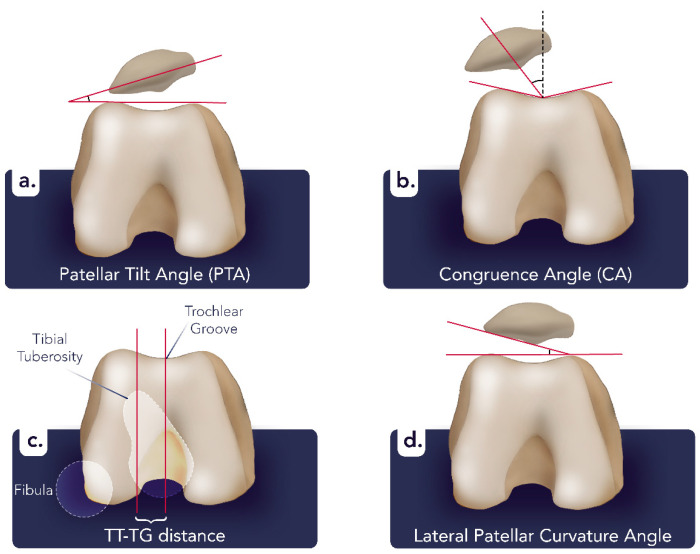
Merchant view of the patellofemoral joint demonstrating key anatomical angles and measurements. Patellar tilt angle (PTA) (a): the angle formed between a line connecting the anterior points of the medial and lateral facets of the patella and a line tangent to the femoral condyles. Normally, it is less than 5° laterally. An increased patellar tilt angle can lead to patellar maltracking. Congruence angle (CA) (b): the angle between a line drawn perpendicularly to the deepest point of the trochlear groove and a line from the same point to the apex of the patella. Normally, it is −6° (within a range from −6° to +16°). A positive CA suggests lateral displacement of the patella, which may be associated with patellar subluxation or lateral tracking issues. Tibial tubercle–trochlear groove (TT-TG) distance (c): the horizontal distance between the lowest point of the trochlear groove and a line connecting the highest points of the medial and lateral femoral condyles, best measured on CT. Generally, a TT-TG distance under 15–20 mm is considered normal. A measurement exceeding this range suggests a lateralized tibial tubercle, which can affect patellar tracking. Lateral patellar curvature angle (LPCA) (d): the angle is formed between a line along the lateral facet of the patella and a perpendicular line to the patella’s axis. While normal values vary, a sharper lateral patellar curvature angle indicates a steeper lateral facet, increasing contact pressures on the lateral patellofemoral joint and contributing to lateral patellar tilt or maltracking. The image was obtained from the University of Washington Medical Imaging database with the required permissions.
